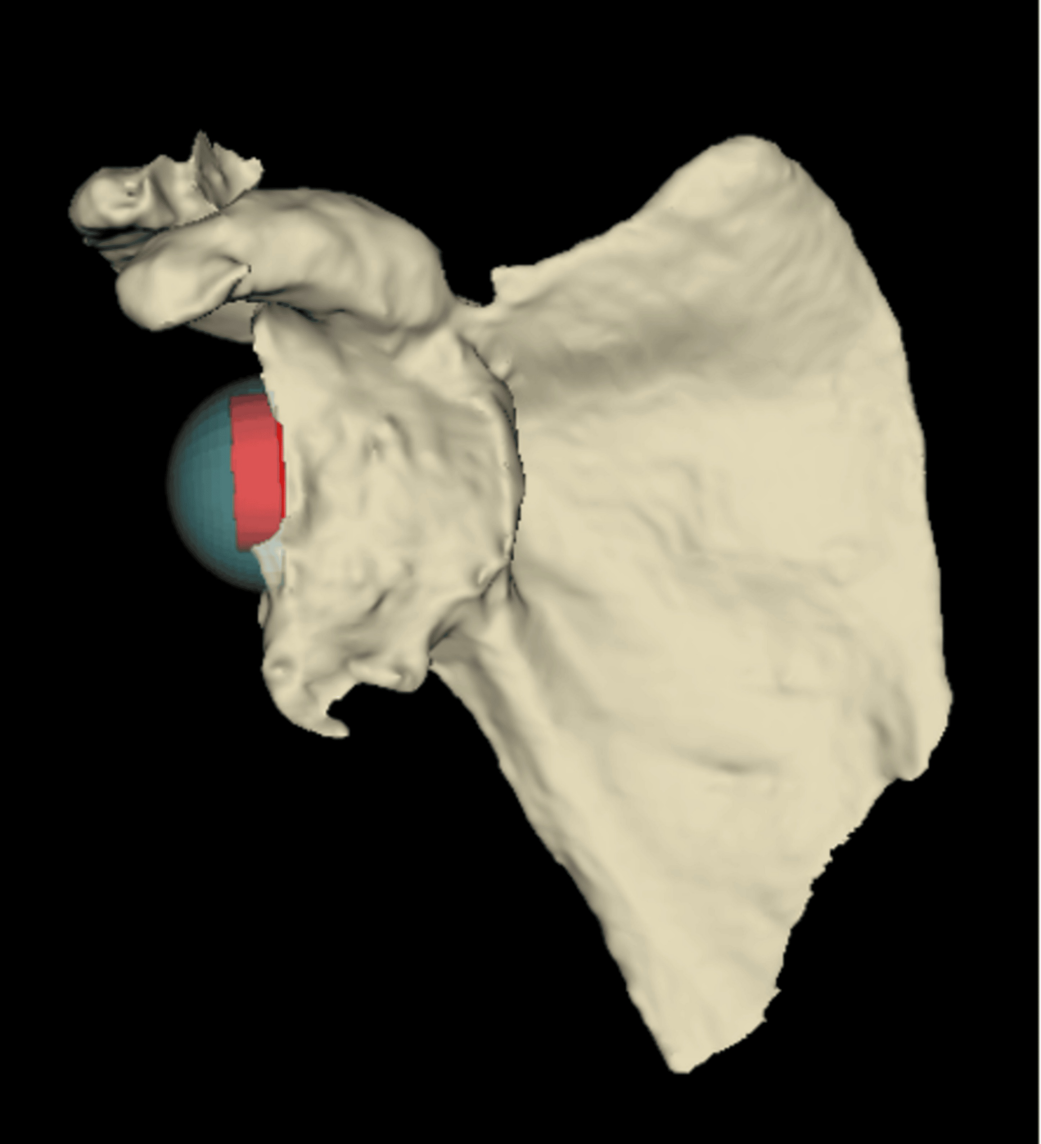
Coronal 3D CT-based planning view demonstrating the glenoid baseplate (size 24) and glenosphere (36 mm).
